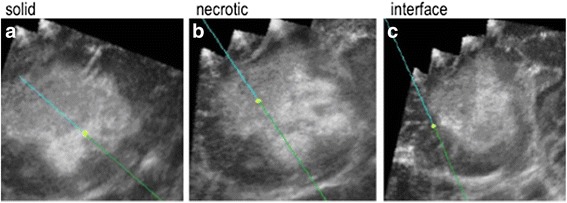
An example of the digital ultrasound images obtained from a WHO grade IV lesion. Biopsies were taken from the solid (a) and nectrotic (b) regions and the infiltrating interface (c) of a WHO grade IV lesion. On these ultrasound images, the *blue line* represents the biopsy needle, whilst the *yellow circle* is the tip, with a projected tip extension shown beyond the tip/yellow circle
